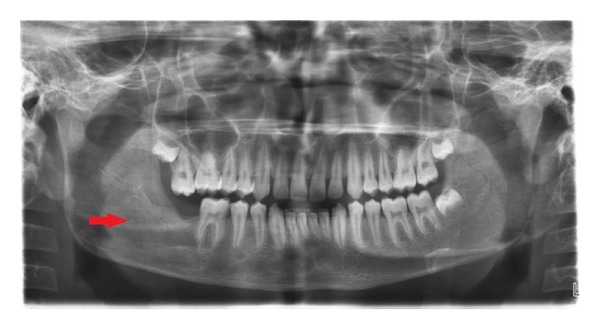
Six-month postoperative orthopantomograph revealed complete bony healing without any recurrence.
